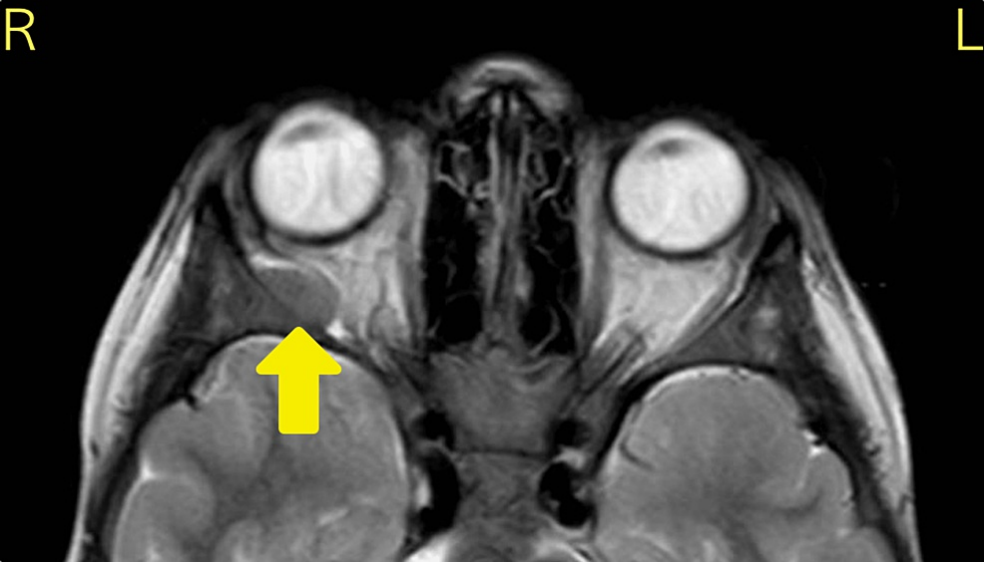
Axial T2 MRI head and orbits for case 2 demonstrated a well-defined, hypointense 12.7x7 mm extraconal mass in the lateral wall of the right orbit (arrow). There is displacement of the right lateral rectus muscle, but no local invasion of other orbital structures.
